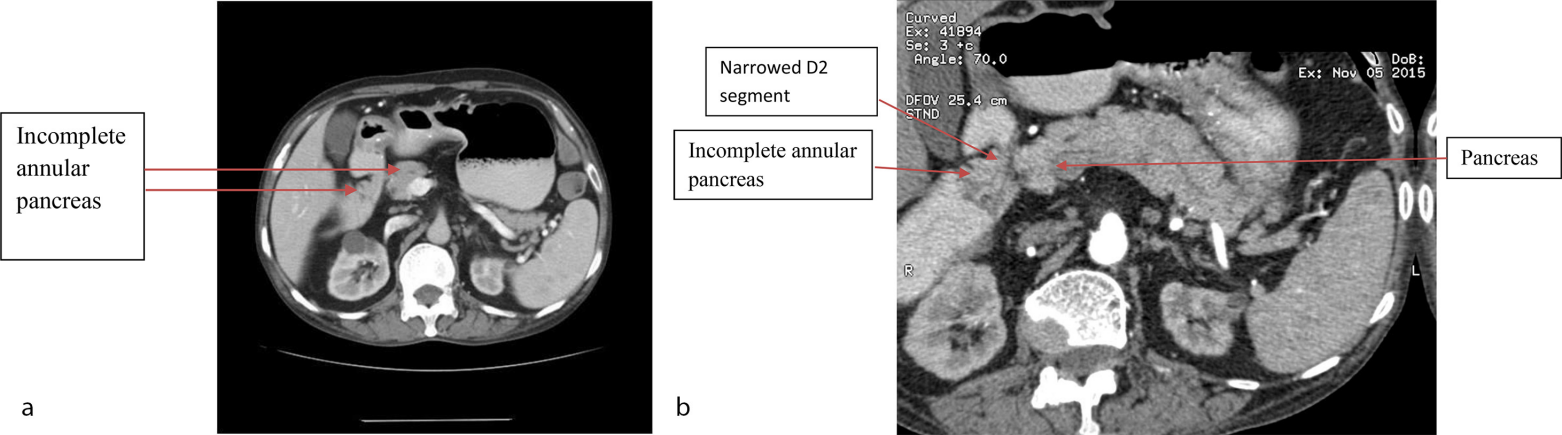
(a) Axial contrast-enhanced CT scan of the abdomen (with oral and intravenous contrast) demonstrating incomplete annular pancreas narrowing the D2 segment of the duodenum. (b) Axial contrast-enhanced CT scan of the abdomen with curved reformatting (with oral and intravenous contrast) demonstrating incomplete annular pancreas narrowing the D2 segment of the duodenum.
